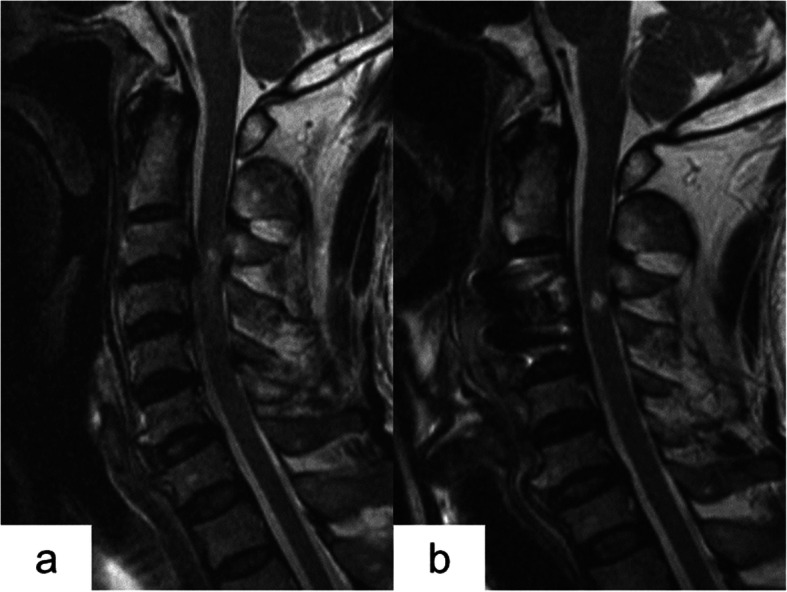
Sagittal magnetic resonance imaging (MRI). a Preoperative MRI showing C3-4 disc herniation and high signal in the spinal cord. b Postoperative spinal cord compression relieved, but MRI showing high signal range of the spinal cord still existing, and the signal becoming stronger
